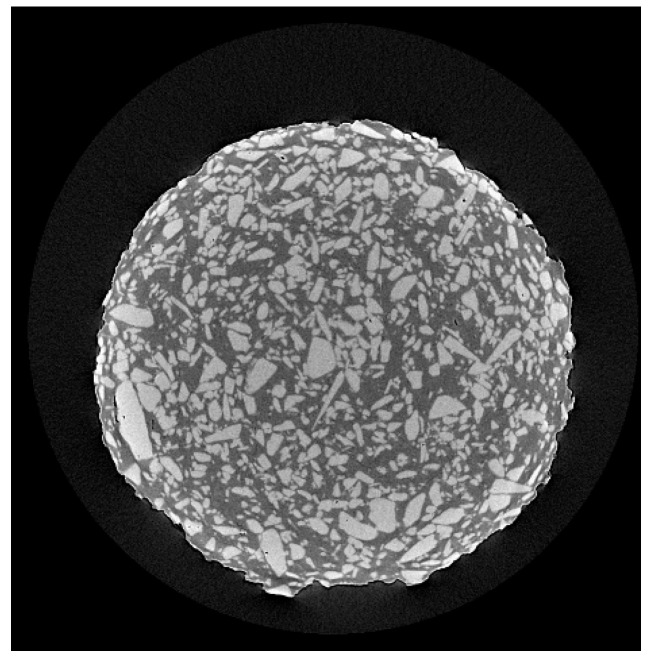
X-ray tomography image of filament with 50% MET, 40% AFF, and 5% of both auxiliary excipients.
